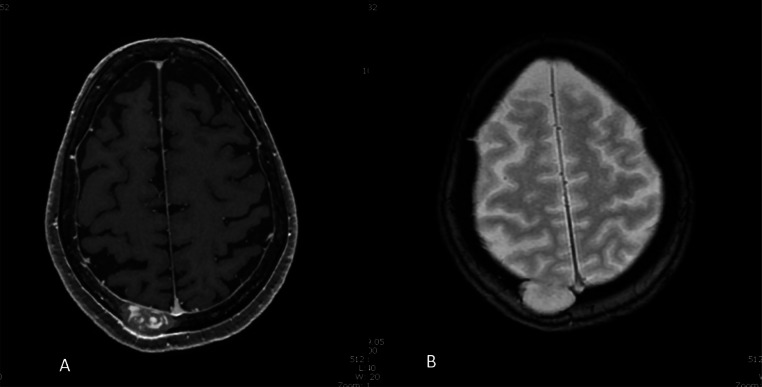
T1-weighted (A) and T2-weighted (B) brain MRI sequences: Osteolytic lesion in the right paramedian posterior parietal vault, eroding the internal and external tables, measuring 34 × 17 mm transverse diameter by 28 mm craniocaudal diameter. In depth, this lesion bulges into the epidural space, and is accompanied by slightly marked neighbouring pachymeningeal contrast. There is no oedematous reaction of the surrounding brain parenchyma.
